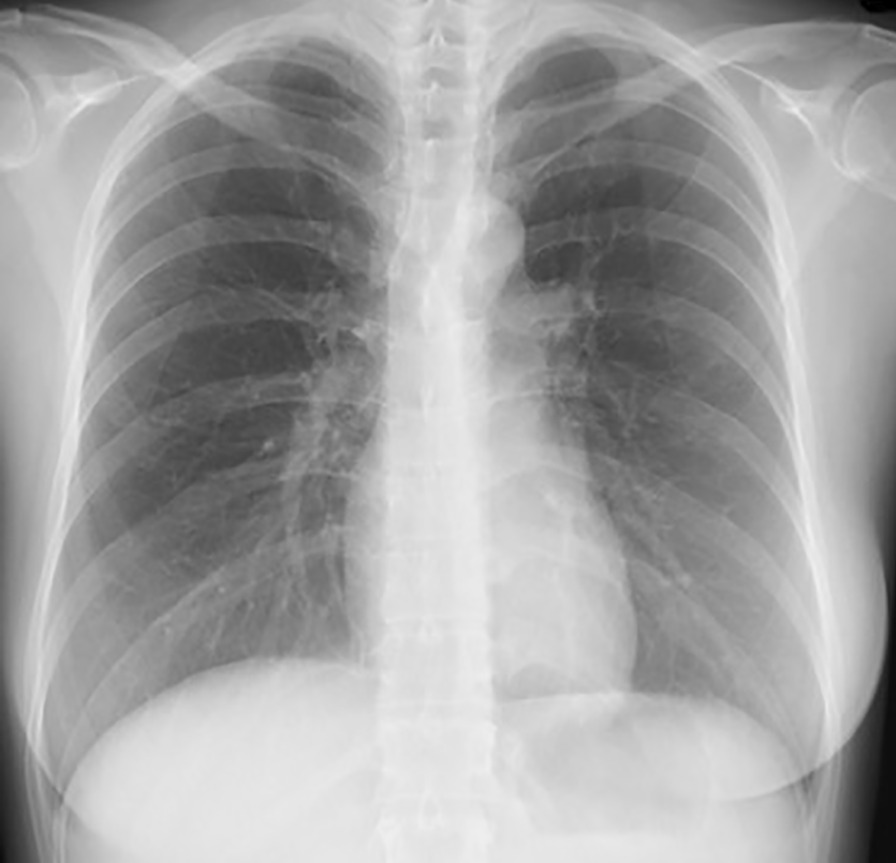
The chest X-ray taken in a standing position 5 days after leaving the intensive care unit. The enlarged pulmonary vascular shadows were improved
